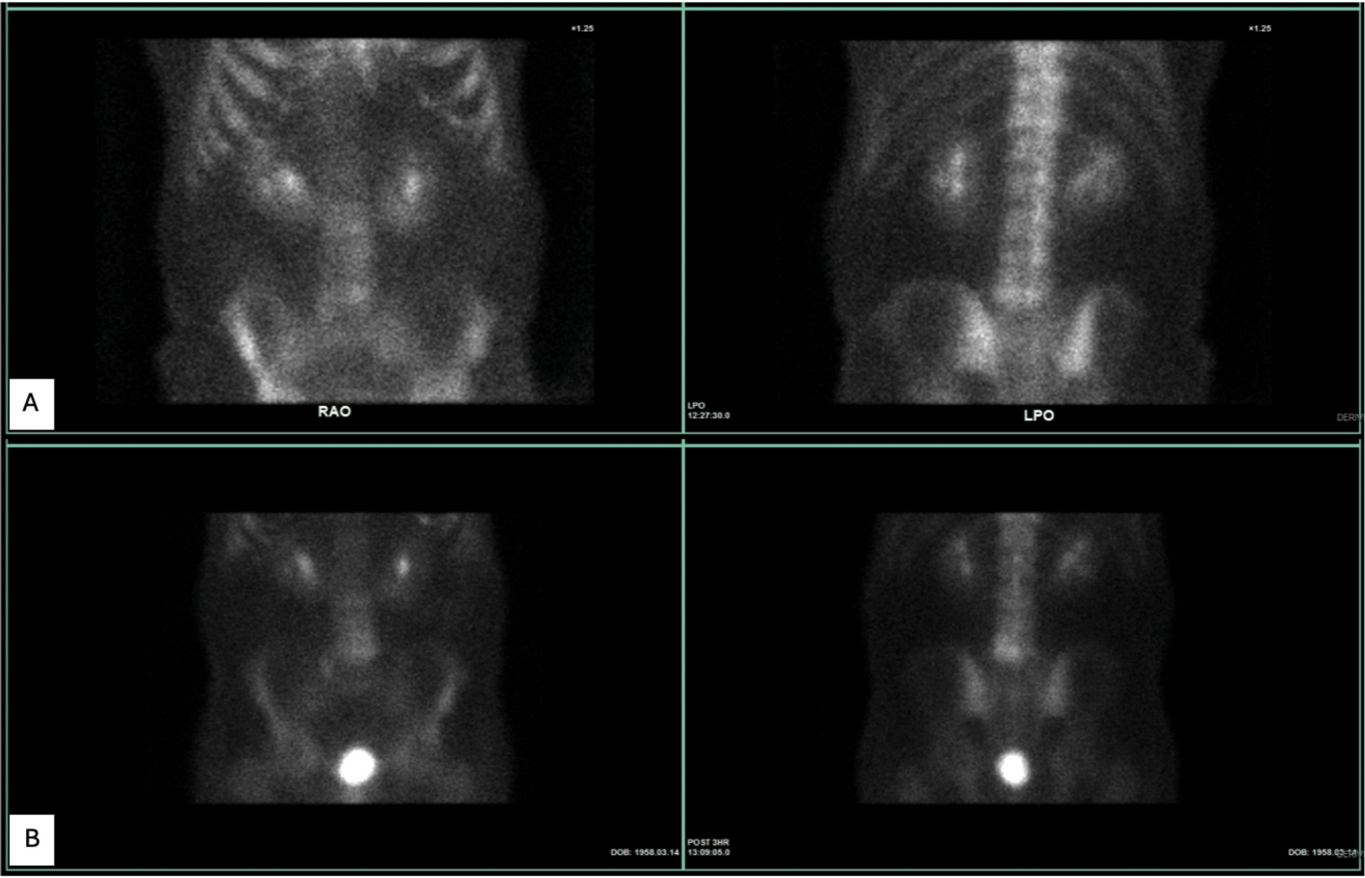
Technetium pyrophosphate nuclear scintigraphy was performed as part of the patient's work-up for the lesion. (A) The first scan performed 32 months after cancer diagnosis, which demonstrated mildly increased uptake in the left side of L5, corresponding to the lesion found on computed tomography in Figure 2. (B) Due to the rather large interval increase in the size of the L5 lesion and the appearance of a new lesion in L4, a repeat scan was performed 47 months after cancer diagnosis, which demonstrated a stable appearance of the mildly increased uptake in L5 identified previously, slightly increased uptake adjacent to this in the inferior margin of L4, and likely non-bridging diffuse idiopathic skeletal hyperostosis in the thoracic spine.
